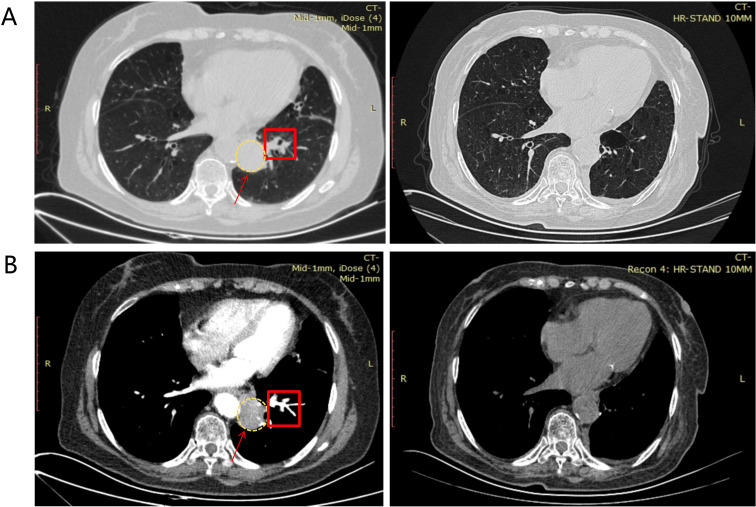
Radiographic evaluation of the patient. (A) Lung window and (B) mediastinal window images. For each panel, the left image is preoperative and the right image is approximately 6 months after initiation of adjuvant ensartinib. The primary lesion is indicated by a red arrow and a yellow dashed circle. Positive lymph node stations (preoperative) are marked by red squares. Postoperative changes following left lower lobectomy and lymph node dissection are observed on the right images, with no measurable residual tumor.
